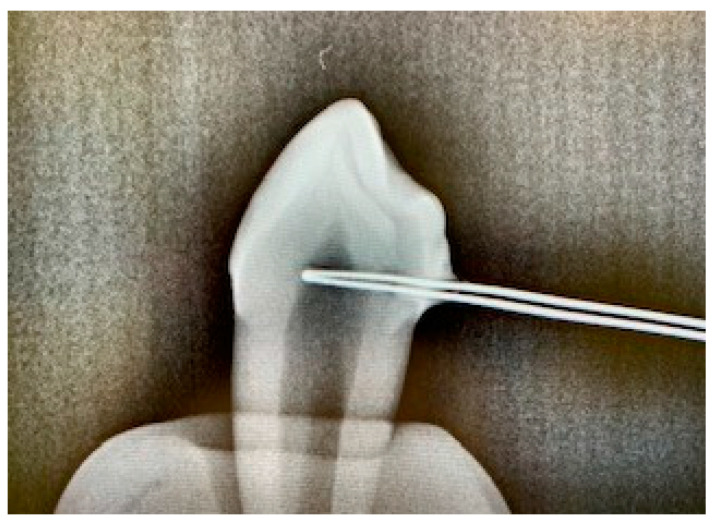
X-ray control image to assess the correct position of the thermocouple.
